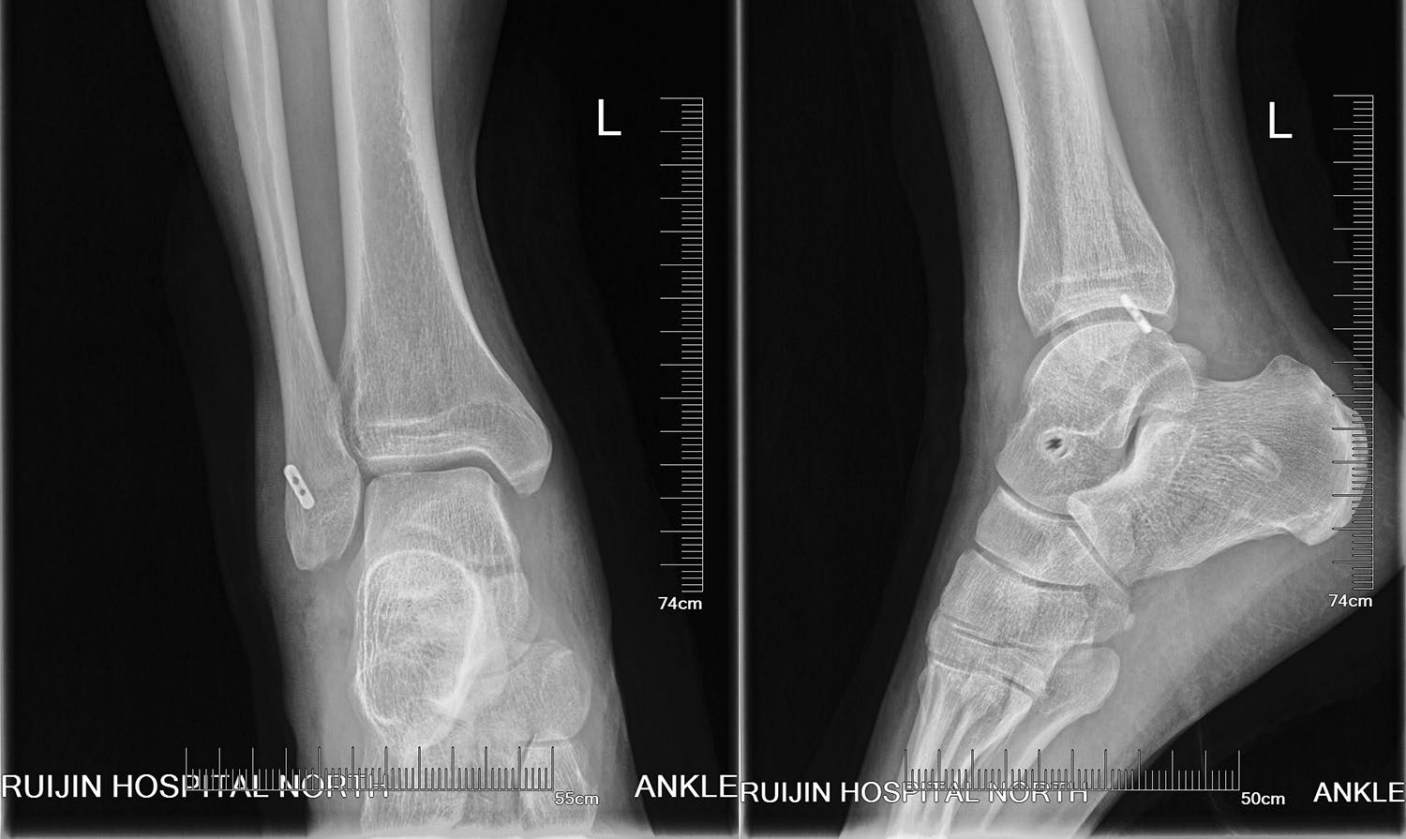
Postoperative radiographic images showing the locations of the cortical button and interference screws
